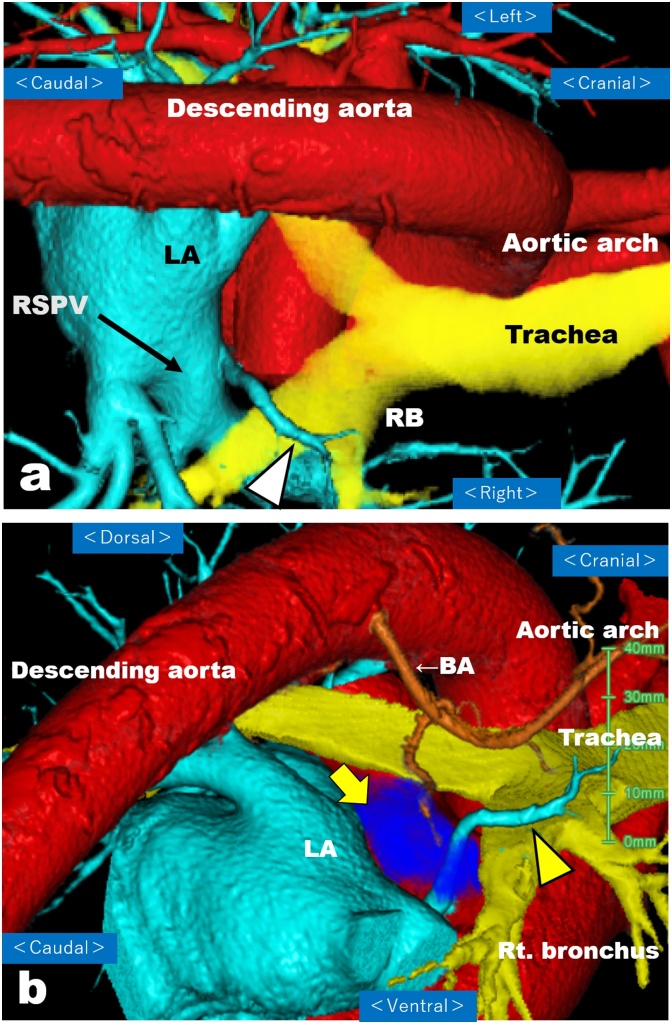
(a) Preoperative reconstructed three-dimensional computed tomography (3D-CT) imaging. The image shows an aberrant V2 (arrowhead) passing behind the right intermediate bronchus and merging with RSPV (arrow). LA: left atrium, RSPV: right superior pulmonary vein, RB: right bronchus. (b) Preoperative reconstructed three-dimensional computed tomography (3D-CT) imaging. The image reveals the aberrant V2 (arrowhead) and branches of the right bronchial artery (thin arrow) running through the subcarinal nodal packet (thick arrow). BA: right bronchial artery, LA: left atrium.
